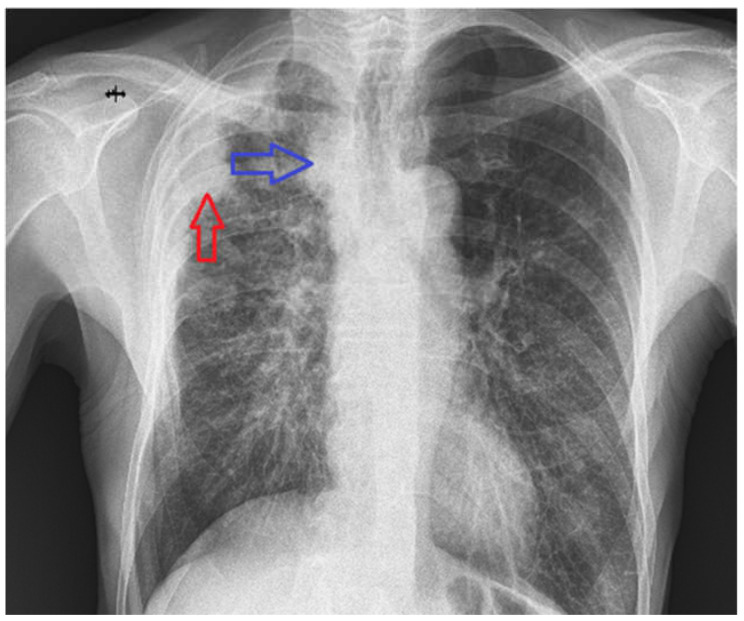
PA chest X-ray—homogeneous opacity, adherent to the right lateral thoracic wall in the upper third and an increased right mediastinal shadow.
